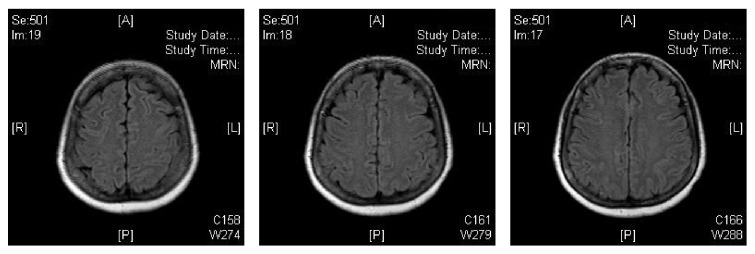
The patient's MRI on admission.
